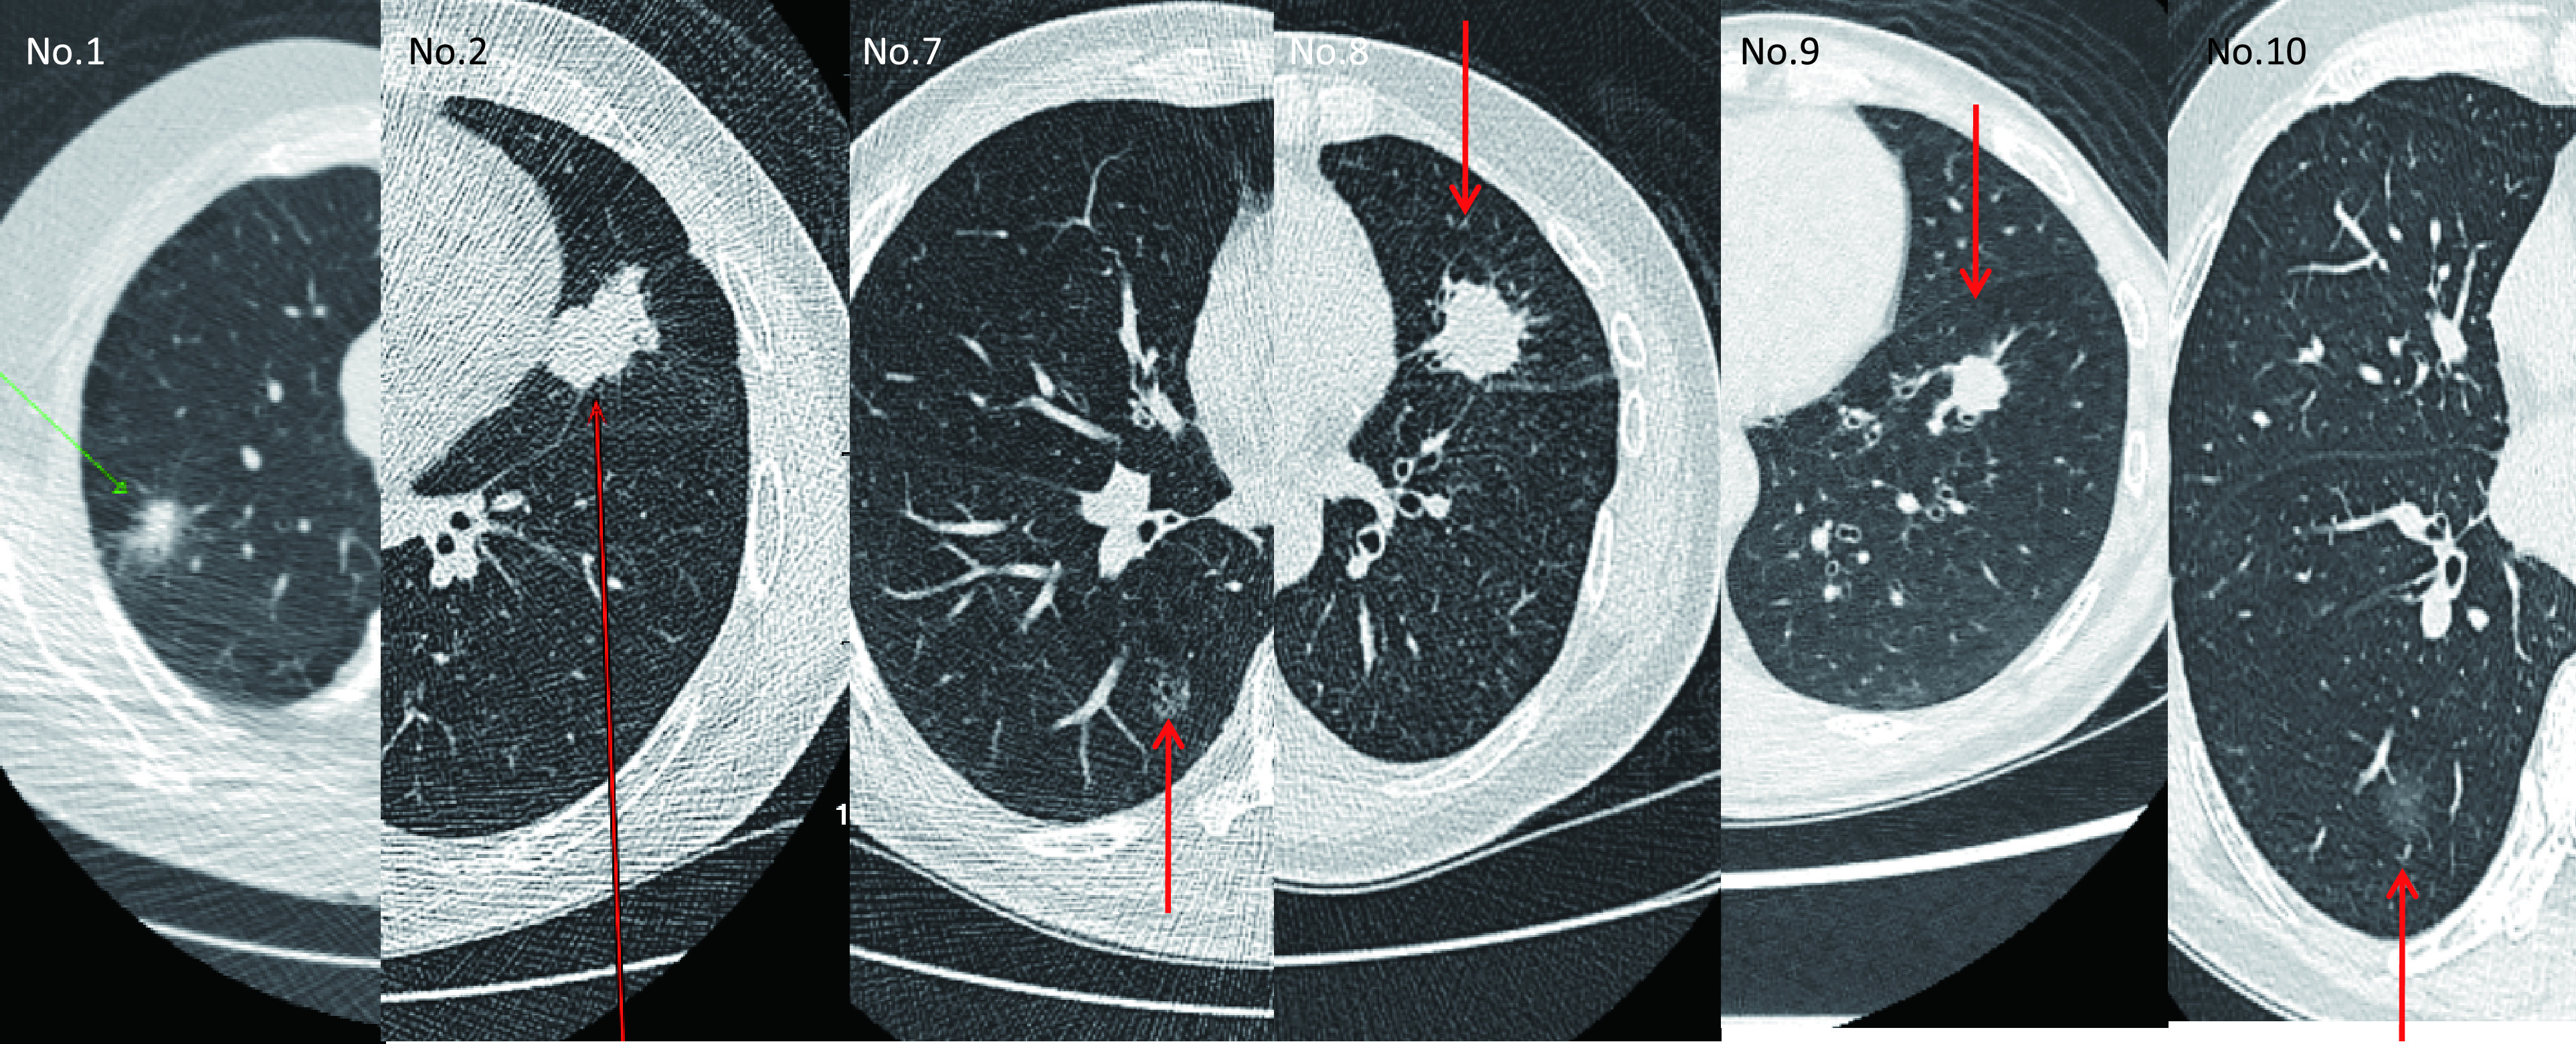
部分肺癌患者CT图。^*^No.：和表 6对应的肺癌患者编号。箭头分别指部分实性结节、实性结节及毛玻璃结节。 CT images of lung cancer patients. ^*^No.: The lung cancer patients'code which corresponds with the Table 6. Arrows point to semi-SN, SN and GGN.
